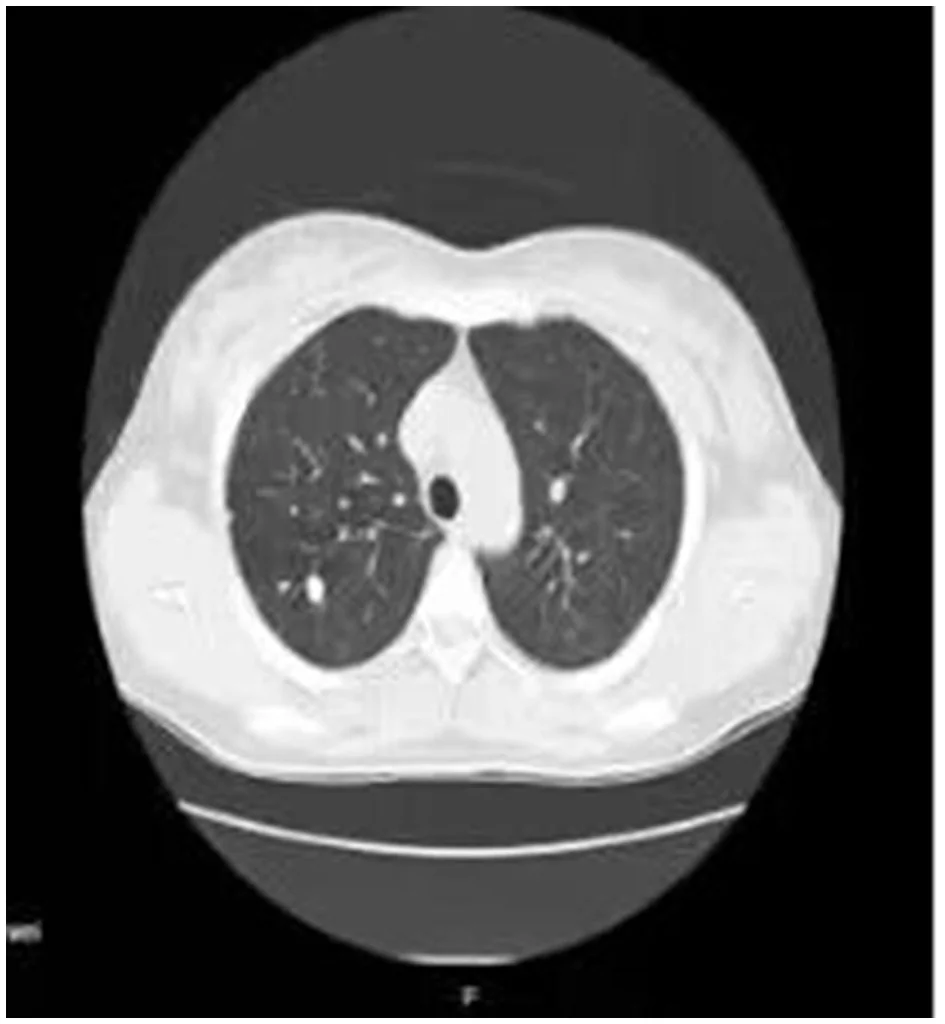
Chest computed tomography of the mother showing multiple nodular shadows.
